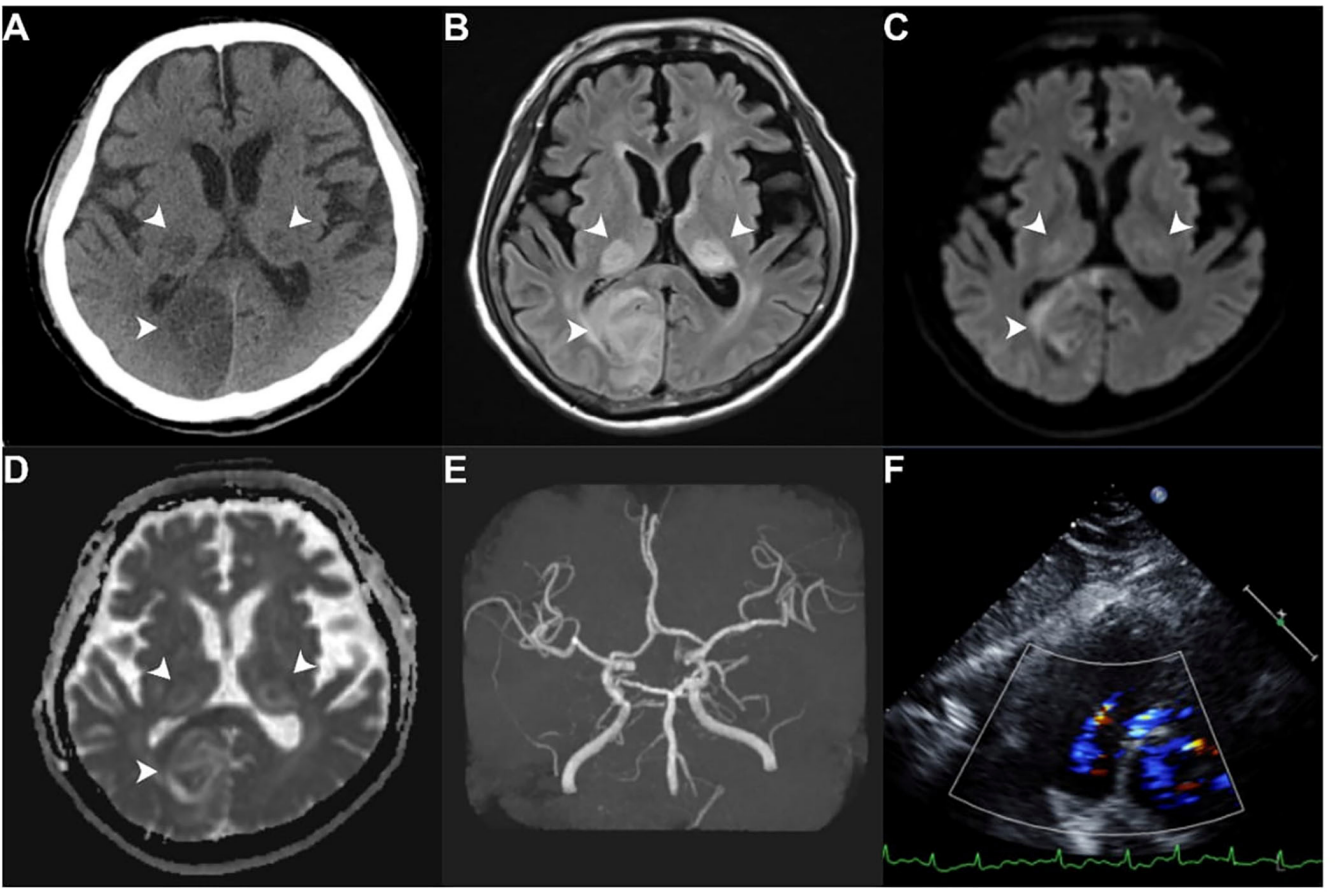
A stroke patient with atrial fibrillation. A 71-year-old male with a history of atrial fibrillation presented with altered mental status. He was admitted to the neurological ward due to a suspected ischemic stroke event. Head computed tomography without contrast revealed hypodense areas in bilateral thalami and occipital lobes [white arrowhead (A)]. Head magnetic resonance imaging confirmed acute cerebral infarction [white arrowhead (B–D)]. Magnetic resonance angiography reported a generalized atherosclerotic change of the bilateral posterior cerebral artery and severe narrowing of the right proximal carotid siphon (E). Transthoracic echocardiography showed a normal left ventricular ejection fraction of 57% (F). (A) Head computed tomography without contrast; (B) T2FLAIR magnetic resonance imaging; (C) Diffusion-weighted magnetic resonance imaging; (D) Apparent diffusion coefficient magnetic resonance imaging; (E) Magnetic resonance angiography; (F) Four chamber echocardiographic views of echocardiography.
